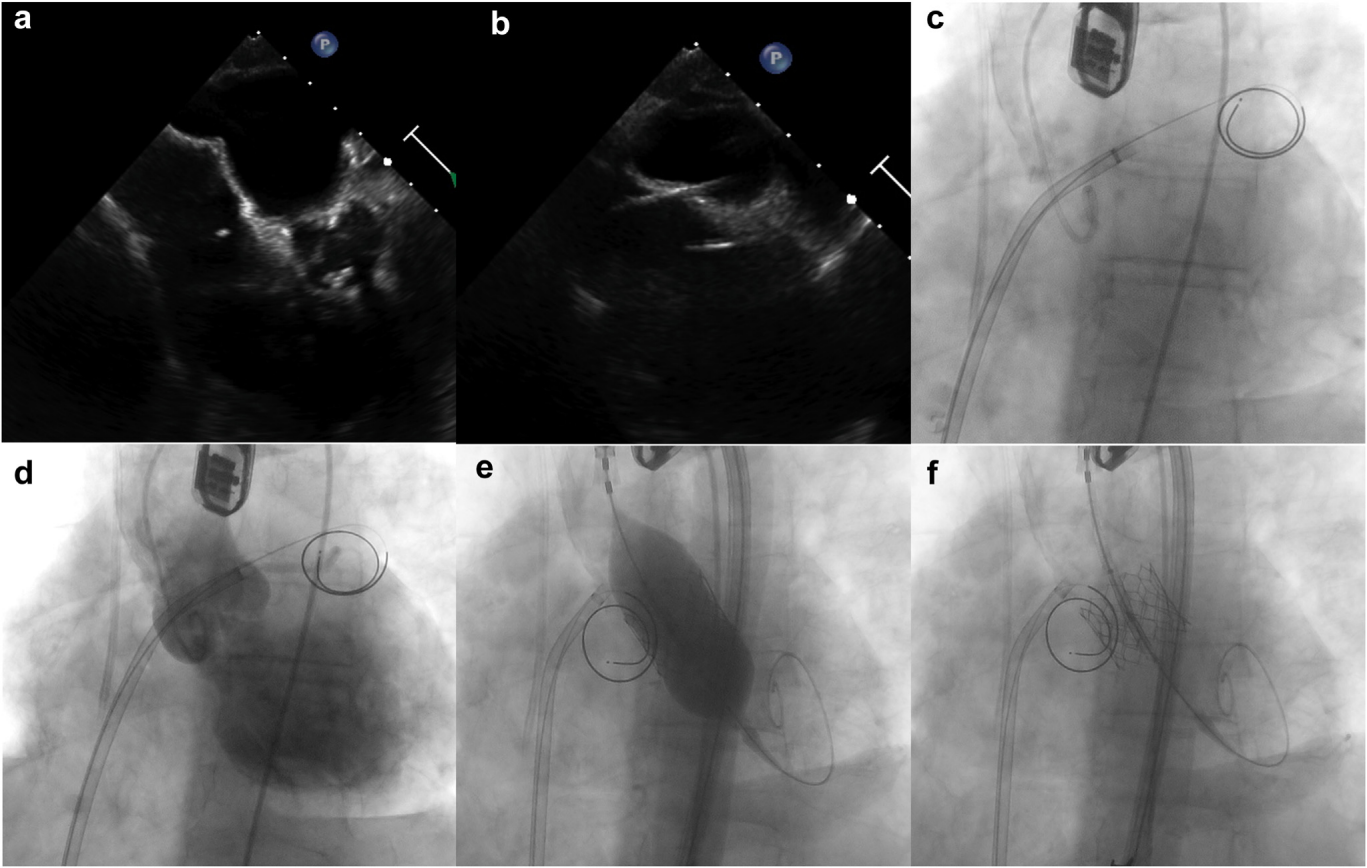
Transeptal puncture and transcatheter aortic valve replacement. (a and b) Transesophageal echocardiography guidance aiming for a posterior and inferior puncture to access the LA. (c) After the transeptal puncture, the VersaCross RF System (Baylis Medical, Montreal, Canada) and the VersaCross RF Pigtail wire (Baylis Medical, Montreal, Canada) were placed in the LA. (d) Aortography confirmed severe aortic regurgitation. (e) Transcatheter aortic valve replacement under rapid pacing via the left ventricle wire. (f) The 23-mm SAPIEN S3 Ultra (Edwards Lifesciences, California, USA) transcatheter heart valve deployed in the aortic position. Abbreviation: LA, left atrium.
